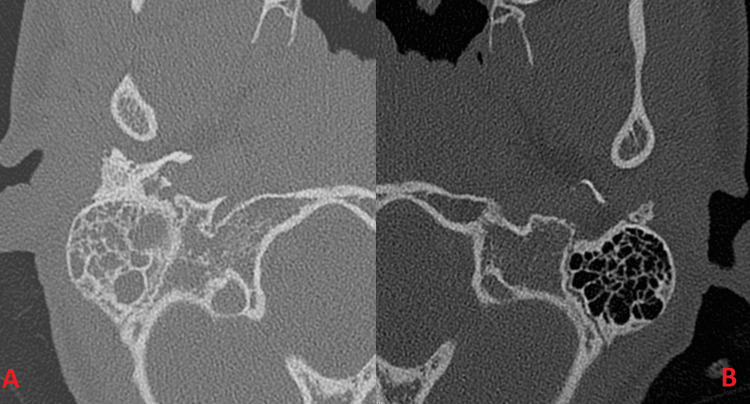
Axial CT head with IV contrast at the level of the mastoid air cells and posterior fossa. The right (A) and left (B) mastoid air cells are pictured. There is opacification of the right mastoid air cells, middle ear, and external auditory canal, which likely represents acute otomastoiditis and otitis externa.
